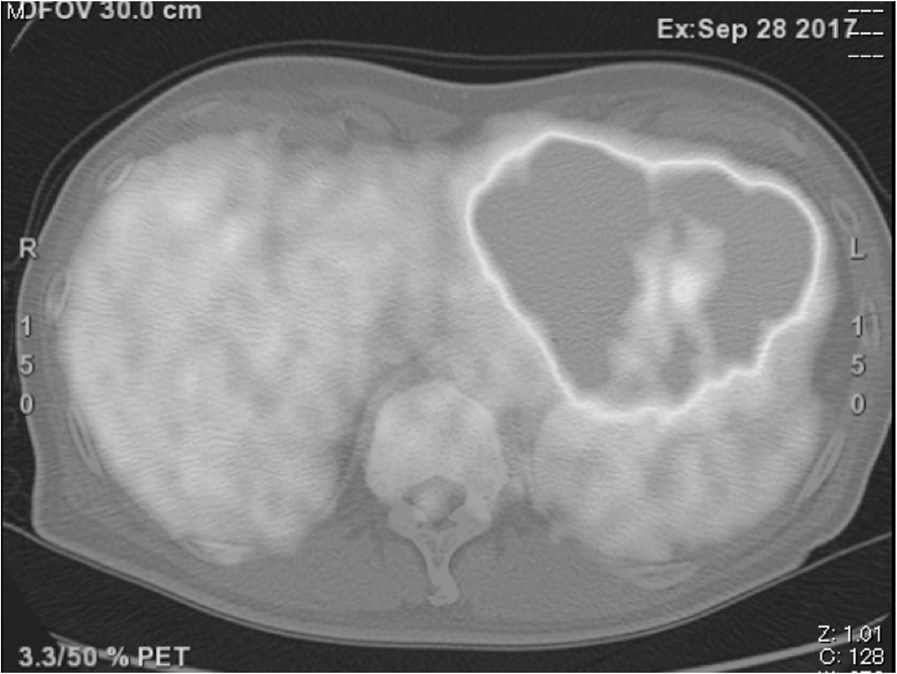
SUVmax of the liver mass in PET was 10.4. No abnormal accumulation was observed in the lymph nodes, bones, or peritoneum
